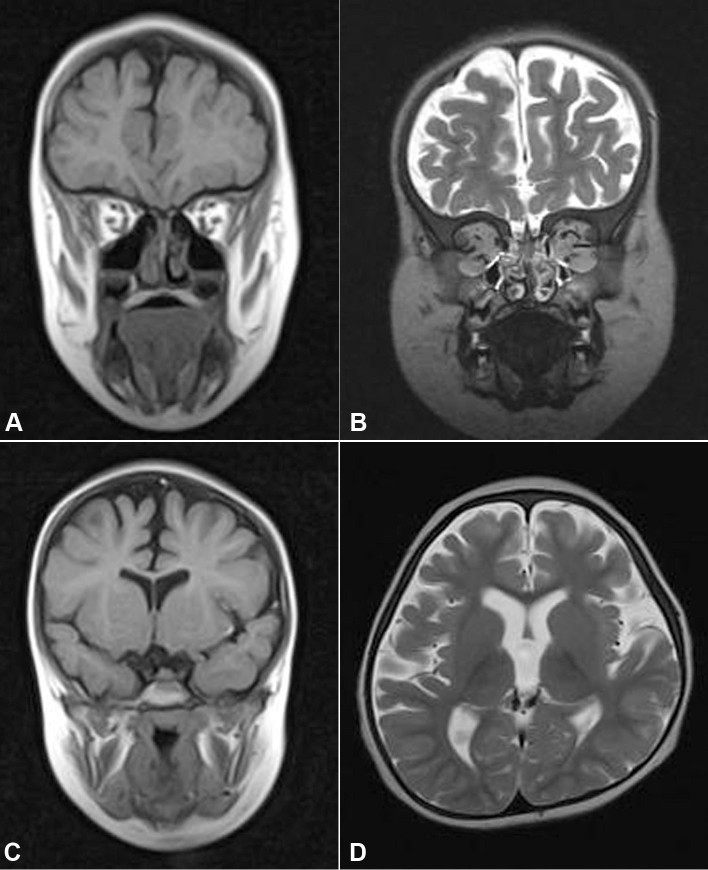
Cerebral and orbital MRI images. A: Family A, Patient IV:5. B: Family B, affected daughter. Orbital hypoplasia. Coronal images, T2-weighted. Agenesis of the eye globes. Rudimentary extraocular muscles. Present and normal lacrimal glands. C: Axial image. T1-weighted. Family A, patient IV:5. Absent frontal sinus. D: Axial image. T2-weighted. Family B, affected daughter. Corticosubcortical atrophy, predominantly in the frontotemporal lobes, with ex vacuo dilation of the ventricles.
